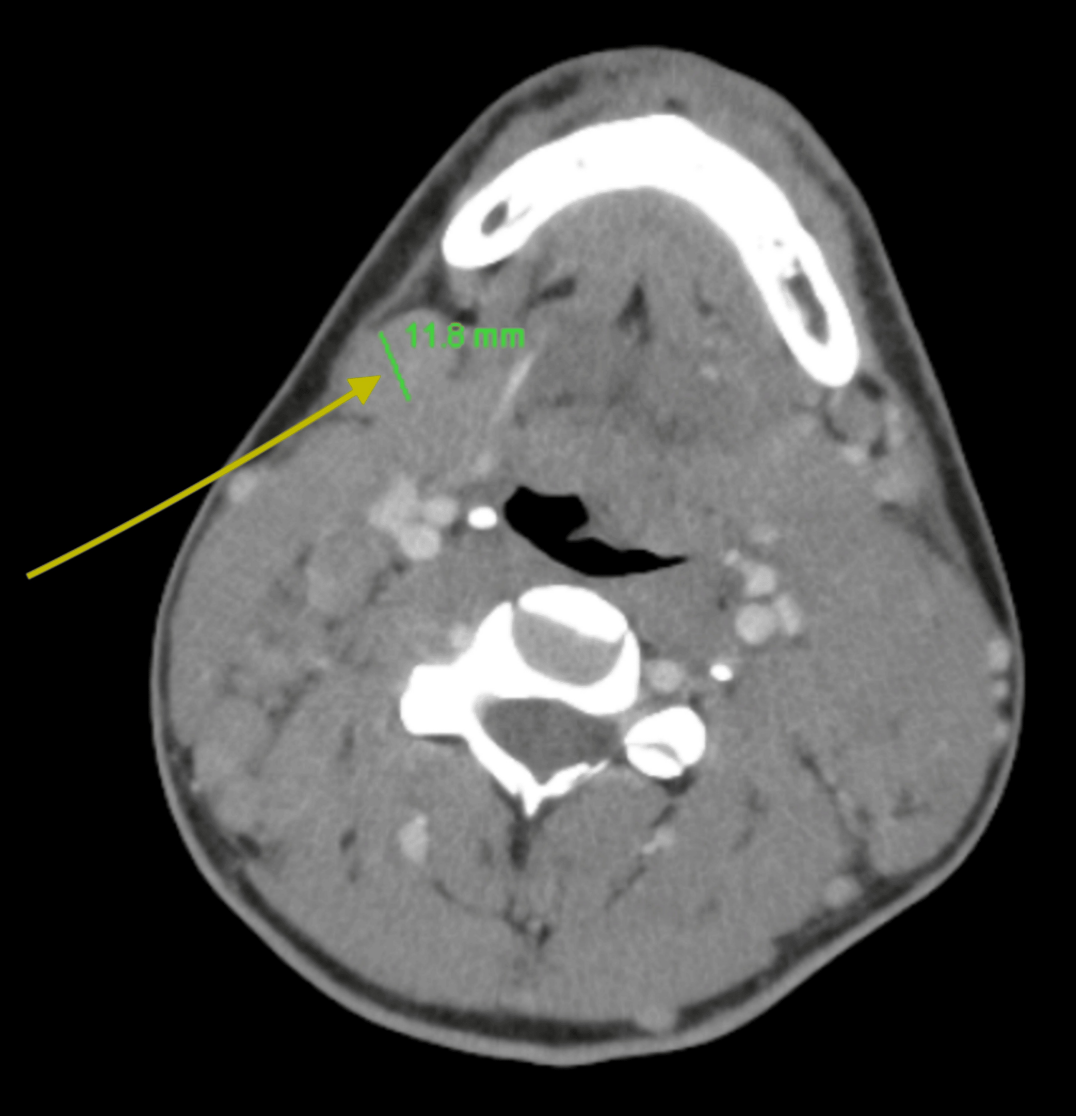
CT of the neck demonstrating enlarged cervical lymph nodes CT: computed tomography
